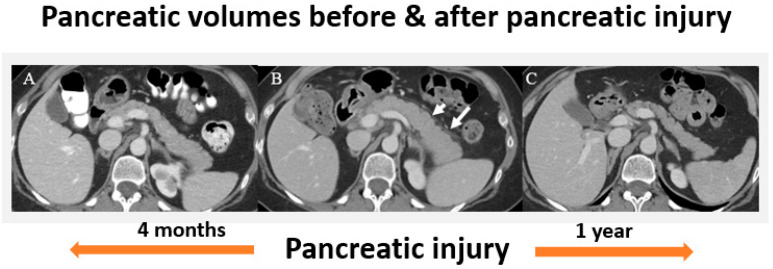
Changes in AIP-3 Over Time on Contrast-Enhanced Computed Tomography (CT). (A) Imaging 4 months before ICI-induced pancreatic injury shows a normally appearing pancreas. (B) At the time of pancreatic injury, there is diffuse enlargement of the pancreas with peripancreatic stranding/edema (arrows). (C) One year after pancreatic injury there is marked pancreatic volume loss without calcification or fatty replacement [15].
